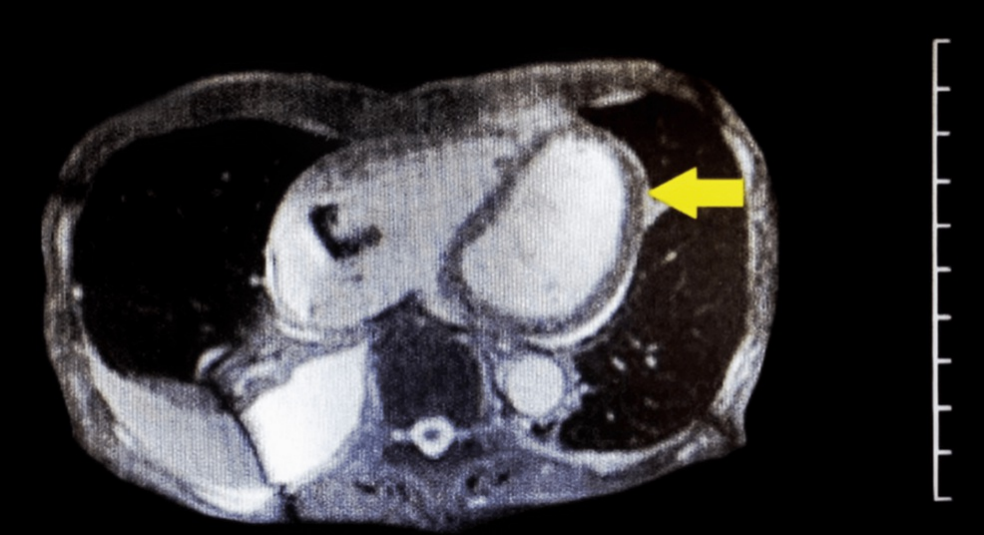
Cardiac MRI axial view showing endocarditis (arrow)
